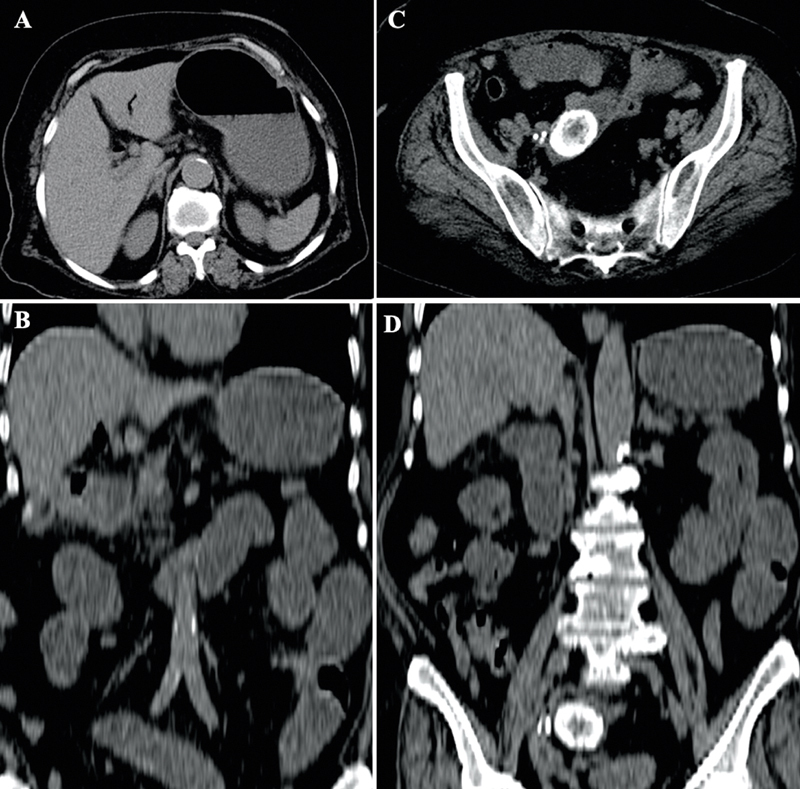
Preoperative CT scan of the abdomen showing pneumobilia (
